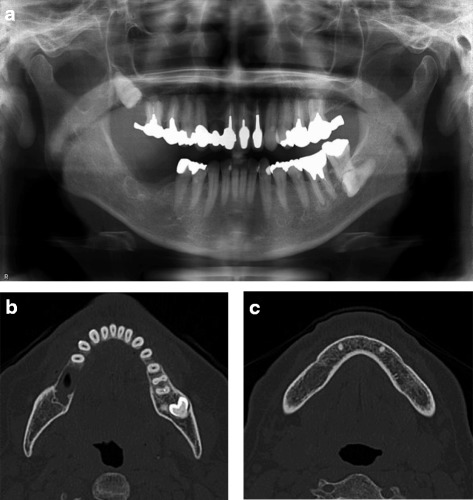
Preoperative a panoramic radiograph and b, c CT image in case 1. Bone resorption was observed in the molar region of the right mandible, and the lesion remained within the mandibular bone
